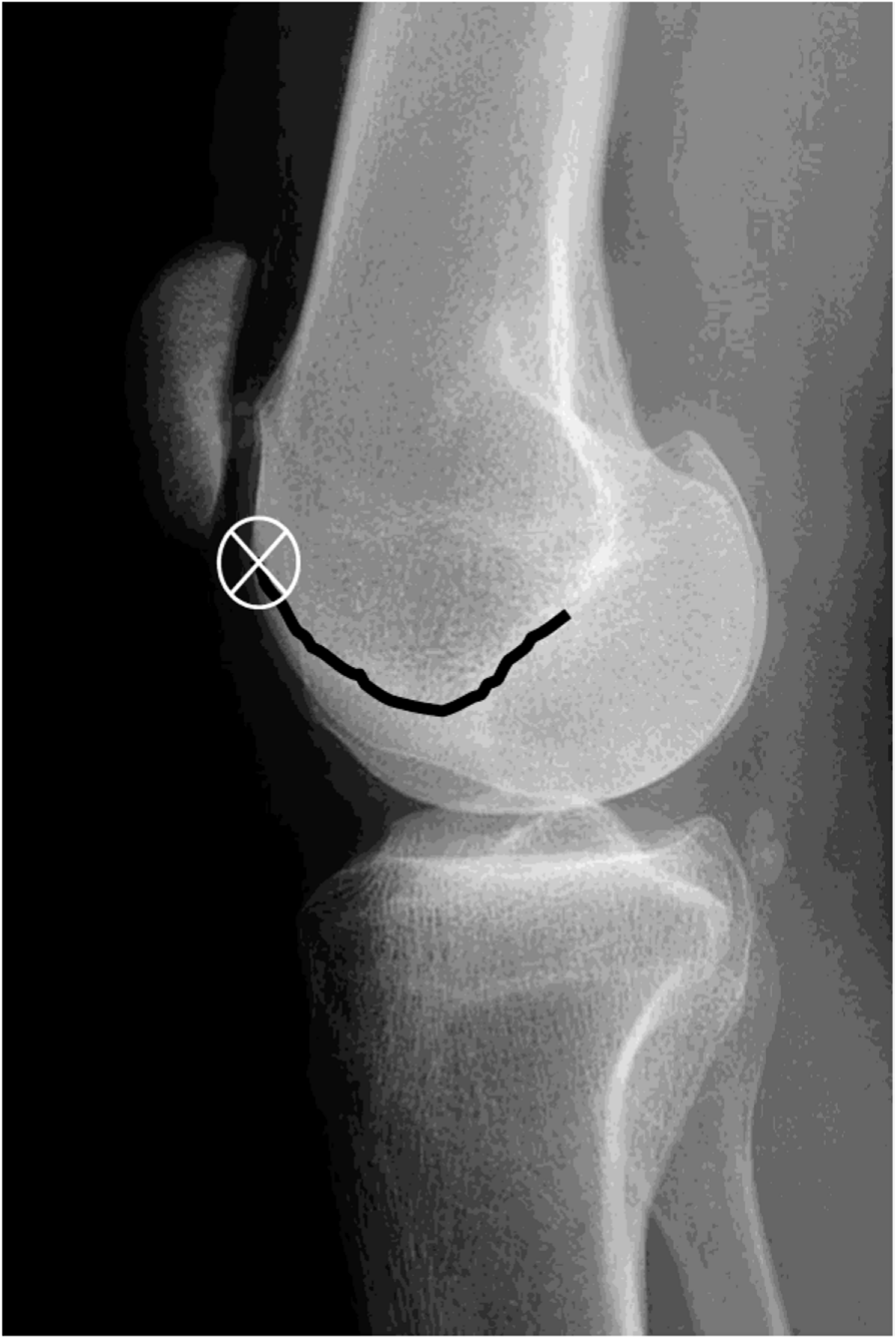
A ‘true’ lateral radiograph of a knee (posterior condyles overlapped). The ‘X’ represents the ‘crossing sign’, where the intercondylar line crosses anterior to the anterior femoral cortex.
